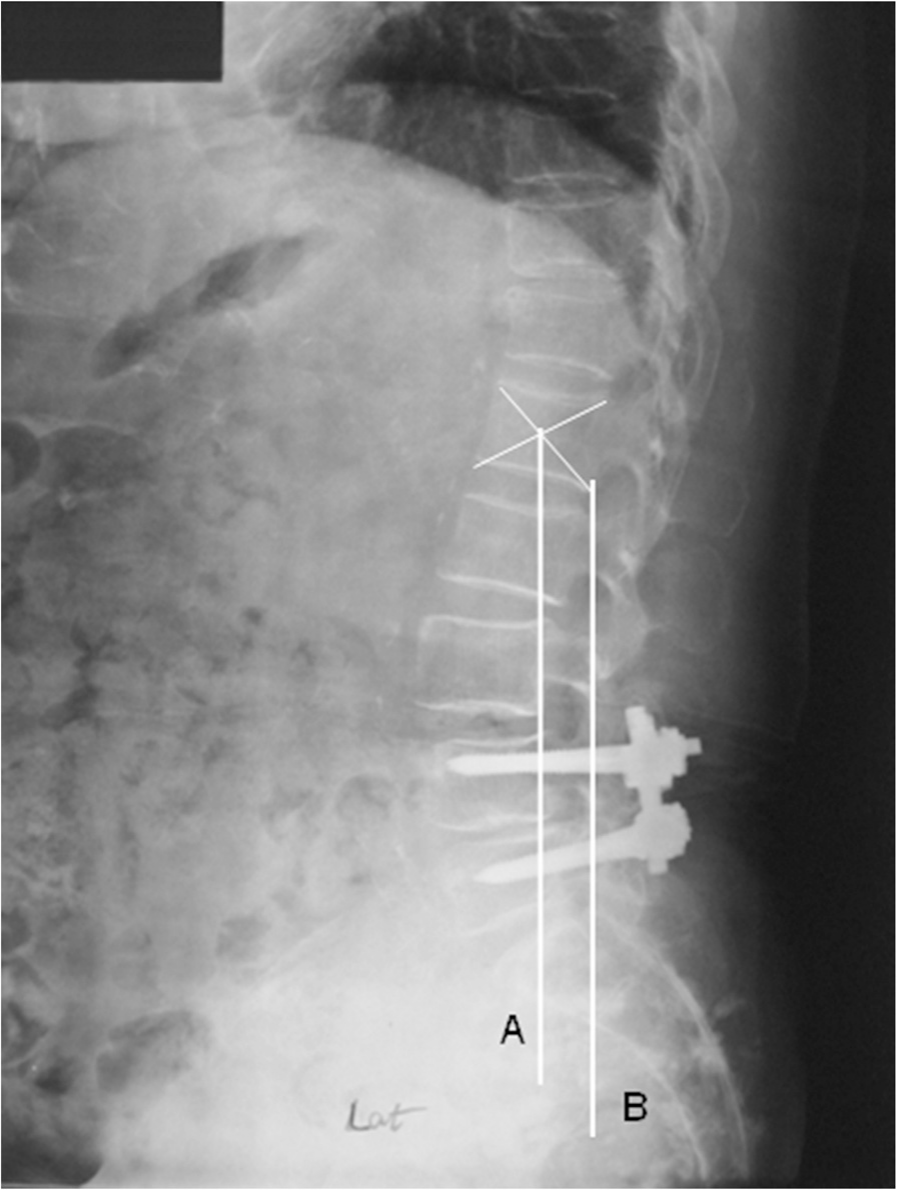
Radiographs were assessed to determine distance from L1 to S1 sagittal plumb line (A–B).
